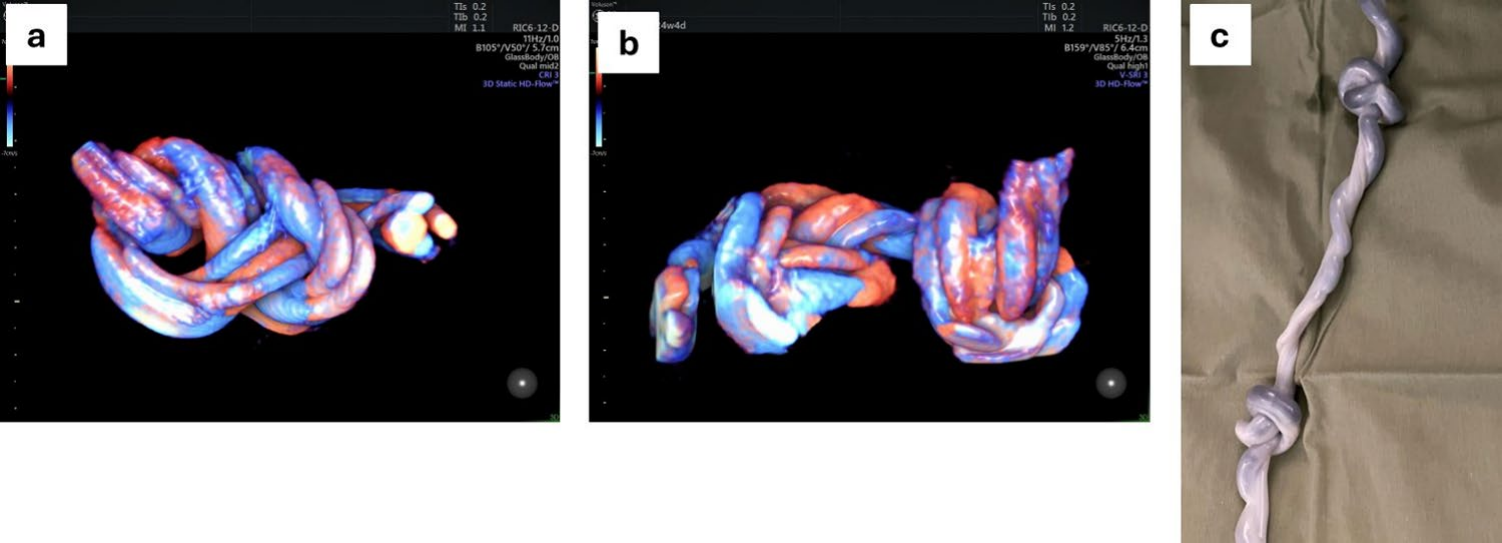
Prenatal and postnatal images of true knot of cord a 3D ultrasound demonstration of a single knot in the umbilical cord b 3D ultrasound demonstration of a double knot in the umbilical cord c postnatal image of a double knot in the umbilical cord
